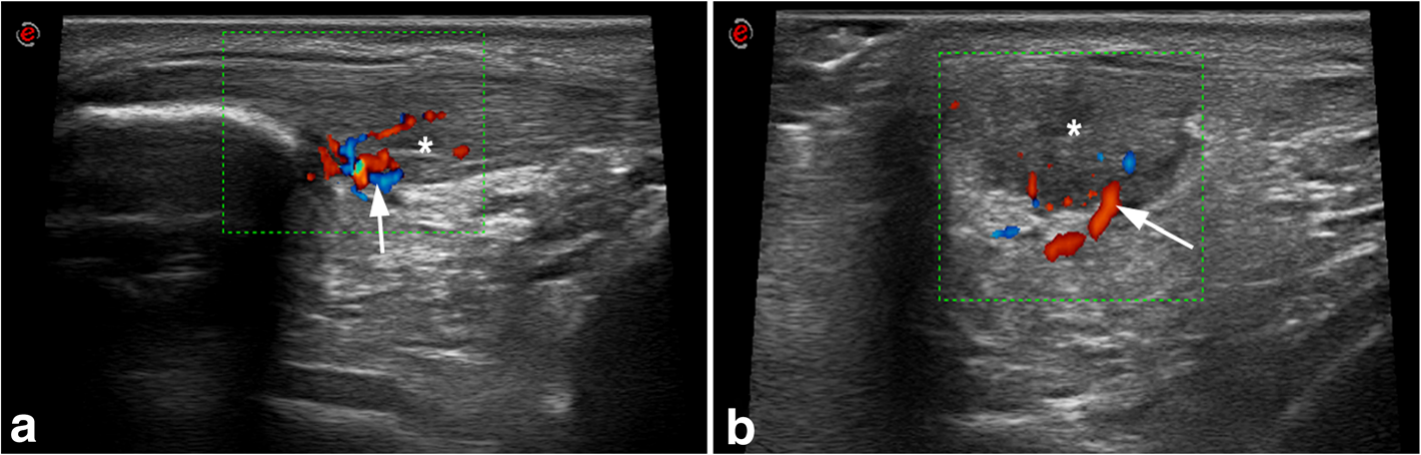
Insertional patellar tendinopathy confirmed with high definition gray-scale US. Longitudinal (a) and transversal view (b) of an injured patellar tendon. The proximal patellar tendon has hypoechoic zones (*) and the color-doppler analysis shows intensive hypervascularization (*arrow*)
